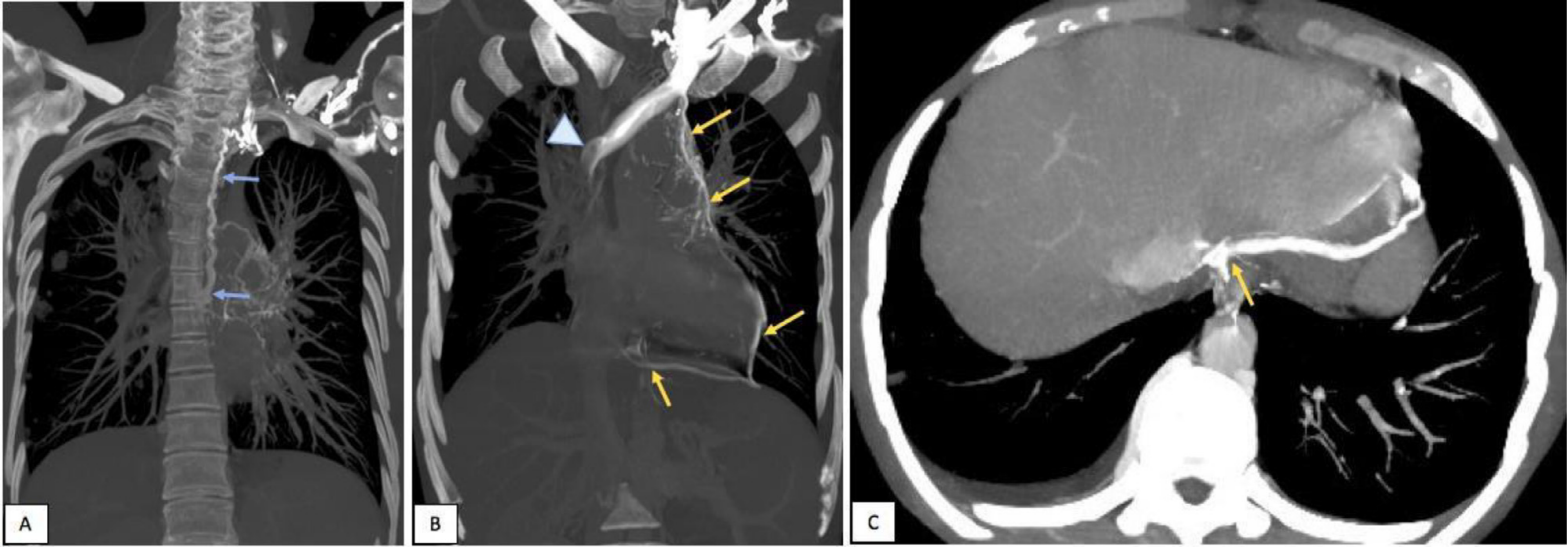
Coronal (A, B) and axial (C) contrast-enhanced CT image with MIP (maximal intensity projection) reconstructions (soft-tissue window); (A) collateral channels draining left brachio-cephalic vein into hemi- azygos which is enlarged (blue arrows). (B, C) collateral channels draining directly into the inferior vena cava (yellow arrows), with thrombosis and abrupt encasement of superior vena cava (Arrowhead).
